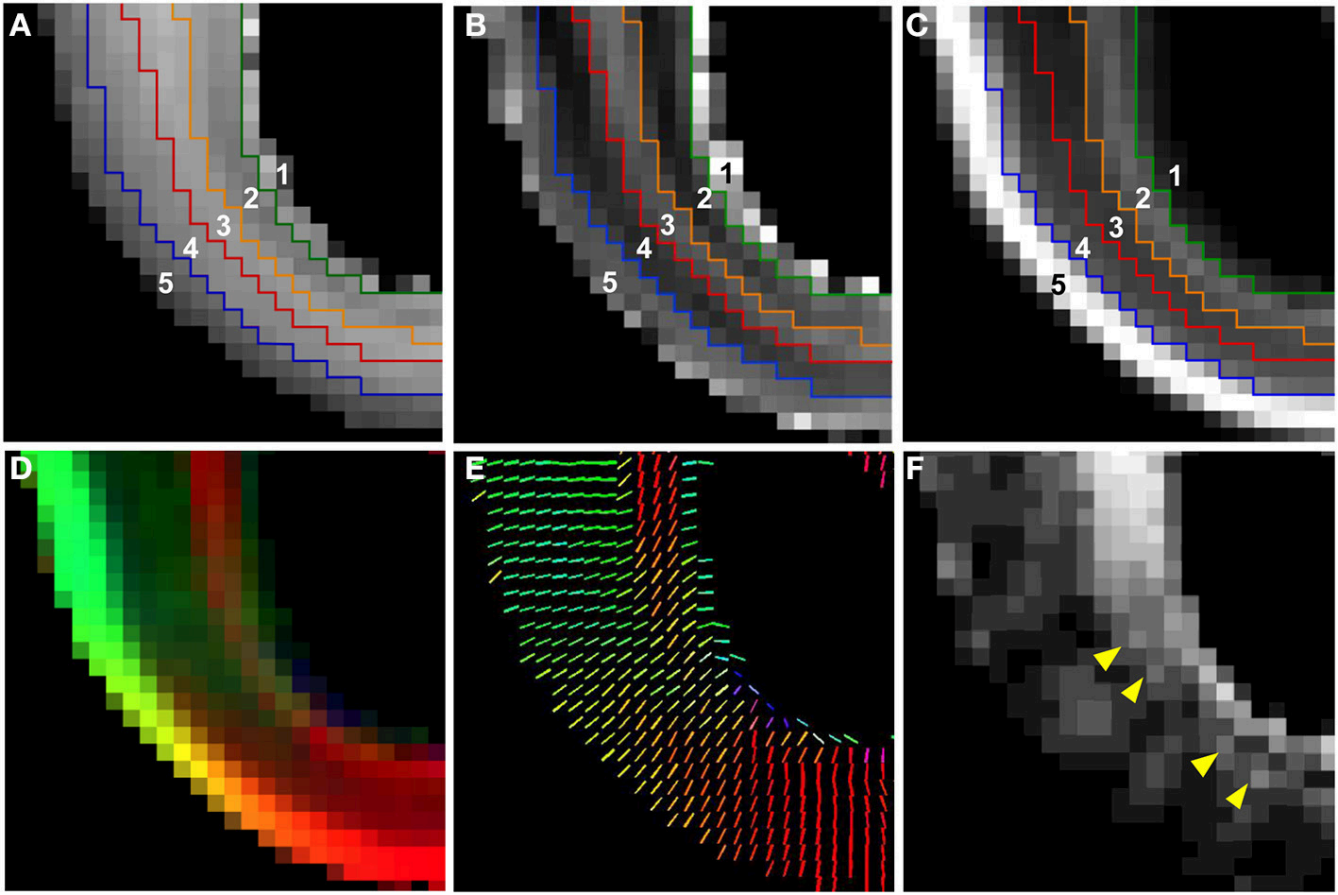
Five tissue zones identified from post mortem MRI. On an enlarged region of the axial views of Figure 4 (Figure 4E, white dashed box), five tissue zones were delineated on ADC maps (B) and the boundaries were projected onto T_2_-weighted image (A) and FA map (C) (see text for details). A diffusion anisotropy color map (D), and a projection of the diffusion tensor primary eigenvector (V_1_) (E) is shown in for the same field of view. For color maps, red, green, and blue channels were scaled by the product of FA and the squared vertical, horizontal, and through-plane component of V_1_, respectively, with the FA map multiplied by a factor of 4. The angle θ between the radial direction vector, and the primary eigenvector of the diffusion tensor is also shown (F). Yellow arrows in (F) indicate locations of local maxima in θ in the superficial part of Zone 2.
